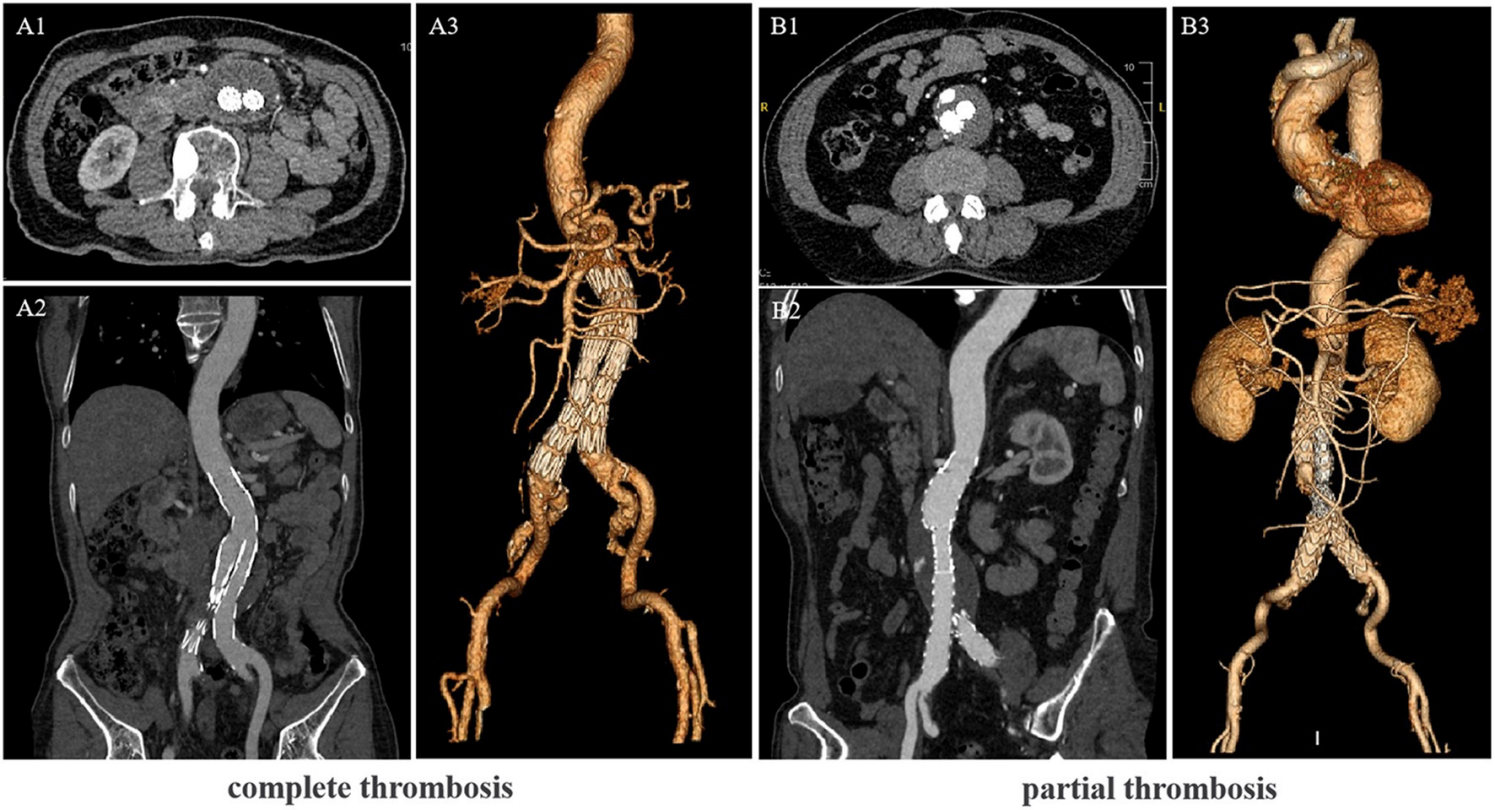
Computed tomography angiography (CTA) scans of the Abdominal aortic aneurysm (AAA) patients after endovascular aortic repair (EVAR). (A) CTA showing the lumen complete thrombosis. (B) CTA showing the lumen partial thrombosis. (A1,B1) Cross-sectional image, (A2,B2) vertical-sectional image and (A3,B3) CTA 3D reconstruction image.
